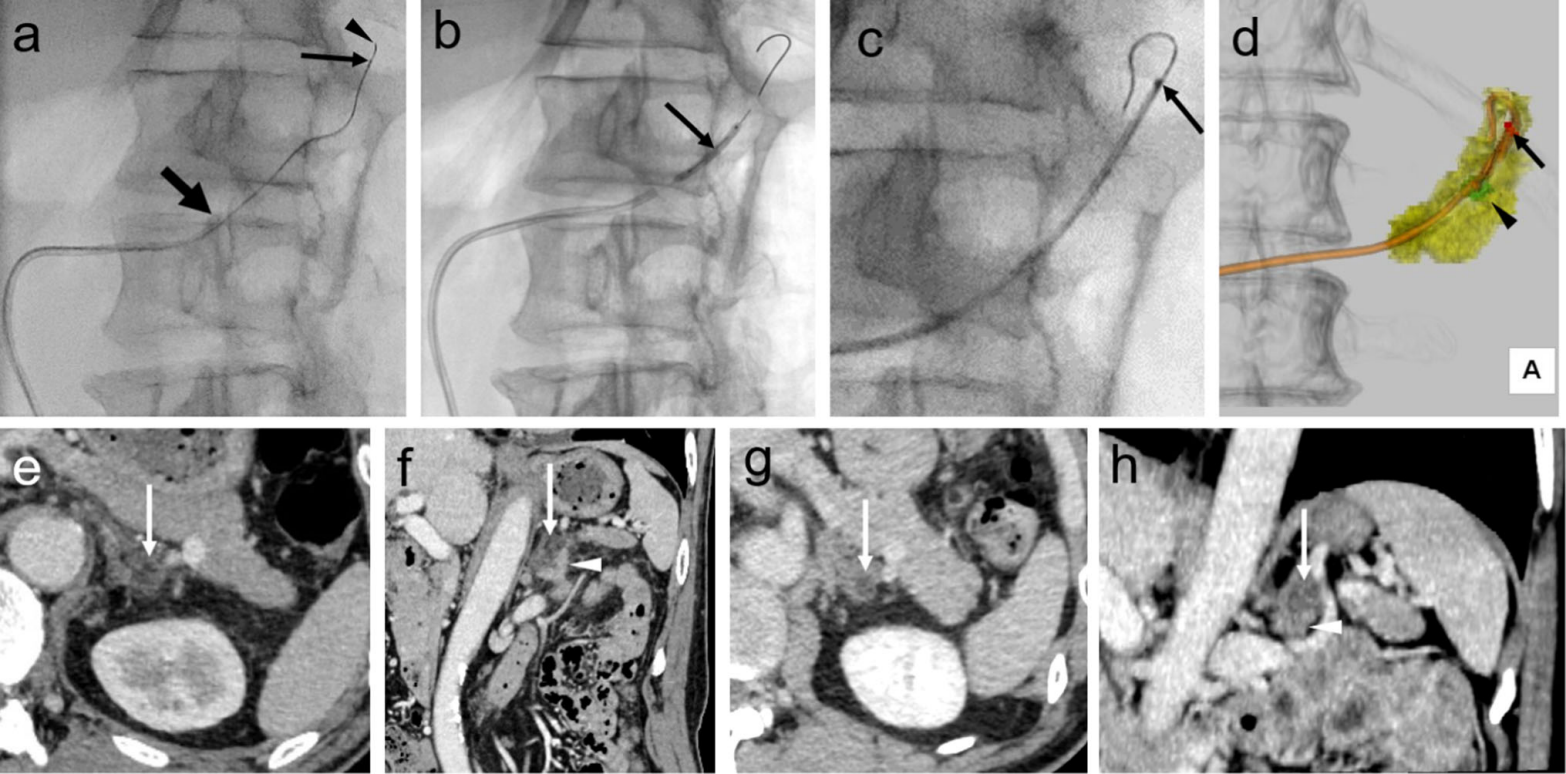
Transvenous catheter radiofrequency ablation of an aldosterone-producing adenoma of the left adrenal gland a The superior lateral tributary vein was catheterized using a 7-Fr guiding catheter (large arrow) inserted into the left adrenal vein, a 1.7-Fr microcatheter (arrow), and a 0.016 inch microwire (arrowhead). b The flexure part of the adrenal tributary vein is dilated using a 2.5 mm diameter, 1.5 cm length balloon catheter (Ikazuchi, KANEKA medical products, Tokyo, Japan) to provide a lumen for insertion of the GOS catheter (arrow). c The GOS catheter was advanced to the end of the superior lateral adrenal tributary vein over the wire (arrow). Then, the catheter tip was pulled back approximately 4 mm by each cauterization, and a total of four cauterizations were performed. The relationship between the catheter tip and the adenoma was not clear in the images, but it was possible to ascertain their relative positions to some extent by comparing the plain CT with the preoperative CT. d Volume rendering image obtained from non-enhanced CT images showing the GOS catheter (orange), tip of the catheter (red) (arrow), adrenal tumor (green) (arrowhead), and adrenal gland (yellow), providing a good view of the relationship between the tumor and catheter tip. e, f After system removal, dynamic CT using 60 mL of iodine-contrast medium was performed. On axial and coronal dynamic CT images, the left adrenal lesion is not detected as an enhanced tumor (arrow). Some edematous changes are seen around the adrenal gland. The lower part of the adrenal gland shows normal enhancement (arrowhead). g, h Five months after the procedure, a dynamic CT for screening of coronal arterial stenosis was performed. While the lower part of the adrenal gland shows enhancement (arrowhead), the left adrenal lesion did not show contrast enhancement (arrow)
